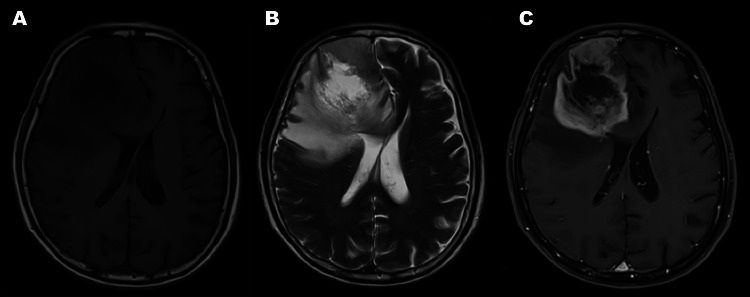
Preoperative MRI (A) Axial T1-weighted MRI showed an irregularly shaped hypointense lesion within the white matter of the right frontal lobe, surrounding a more hypointense core, which was hyperintense on T2-weighted imaging (B), with surrounding vasogenic edema, which exerts a mass effect to the adjacent medial frontal gyri and the frontal horn of both lateral ventricles. (C) A contrast study showed a sizeable mass exhibiting peripheral enhancement and central non-enhancement, which was indicative of necrosis. MRI: magnetic resonance imaging
